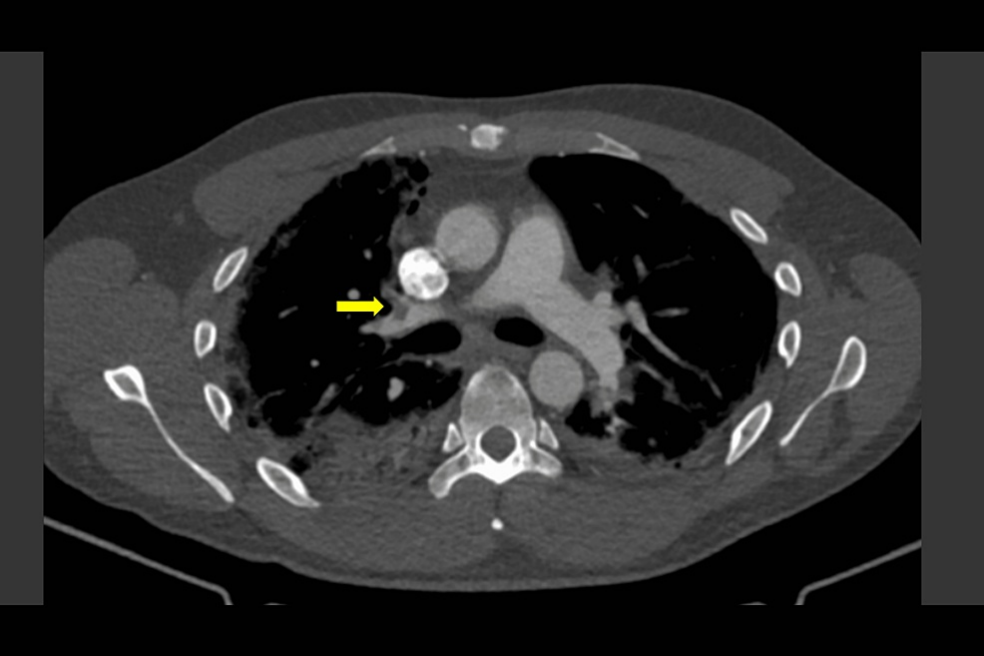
CTPA day 9: axial slice in the bone window showing a right segmental pulmonary embolism (yellow arrow) in situ CTPA, computed tomography pulmonary angiogram
